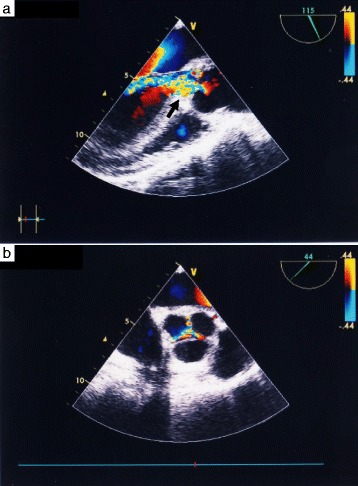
Preoperative transesophageal echocardiography (just after the induction of systemic anesthesia). a Moderate and wide aortic regurgitation can be seen (*arrow*). b The shape of the aortic valve was tricuspid
